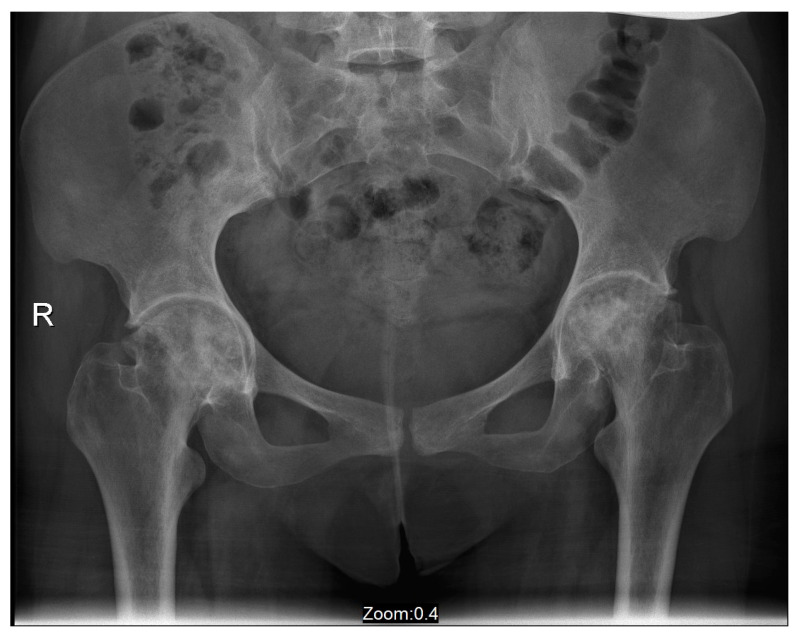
An antero-posterior X-ray of pelvis and hip joints of Case 1 performed four years after the acute events. These findings present severe bilateral osteoarthritis.
